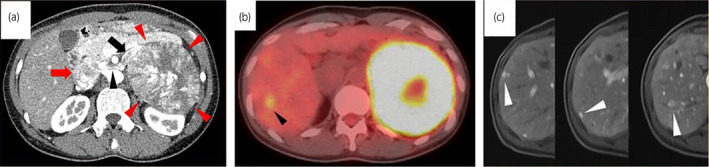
(a) Contrast‐enhanced CT showed a 110 × 100‐mm left adrenal mass (red arrowheads) and tumor thrombus extending to the IVC (black arrow: left adrenal vein, black arrowhead: left renal vein, red arrow: IVC). (b) PET‐CT showed a liver metastasis (black arrowhead), and the SUVmax was 3.7. (c) MRI showed multiple liver metastases (white arrowheads).
